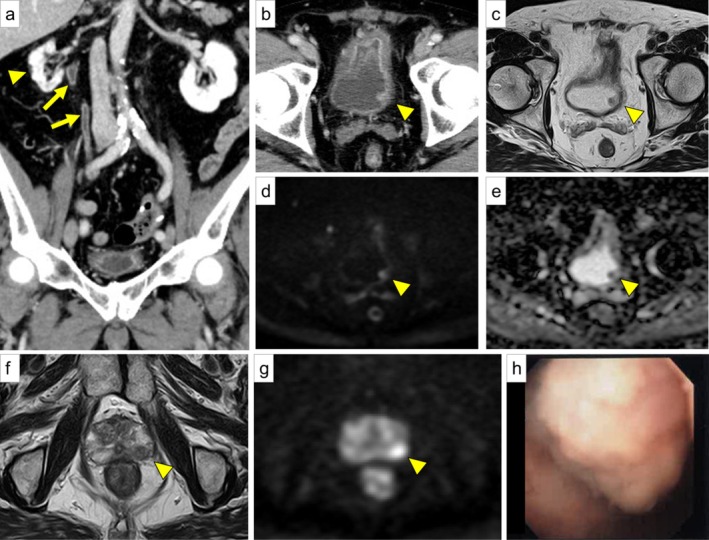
Imaging and cystoscopic findings at initial presentation. (a, b) Contrast‐enhanced abdominal CT; (a) right renal atrophy (arrowhead) and right ureteral wall thickening (arrow). (b) Suspected tumor on the left lateral bladder wall (arrowhead). (c–e) Bladder MRI; (c) T2WI, (d) DWI, and (e) ADC map. Suspected non–muscle‐invasive clinical T1 bladder cancer (arrowhead). (f, g) Prostate MRI; (f) T2WI and (g) DWI. A PI‐RADS v2.1 category 4 lesion suspicious for prostate cancer in the dorsal peripheral zone of the left lobe (arrowhead). (h) Cystoscopy showing a non‐papillary mass on the left lateral bladder wall (limited by cloudy urine).
